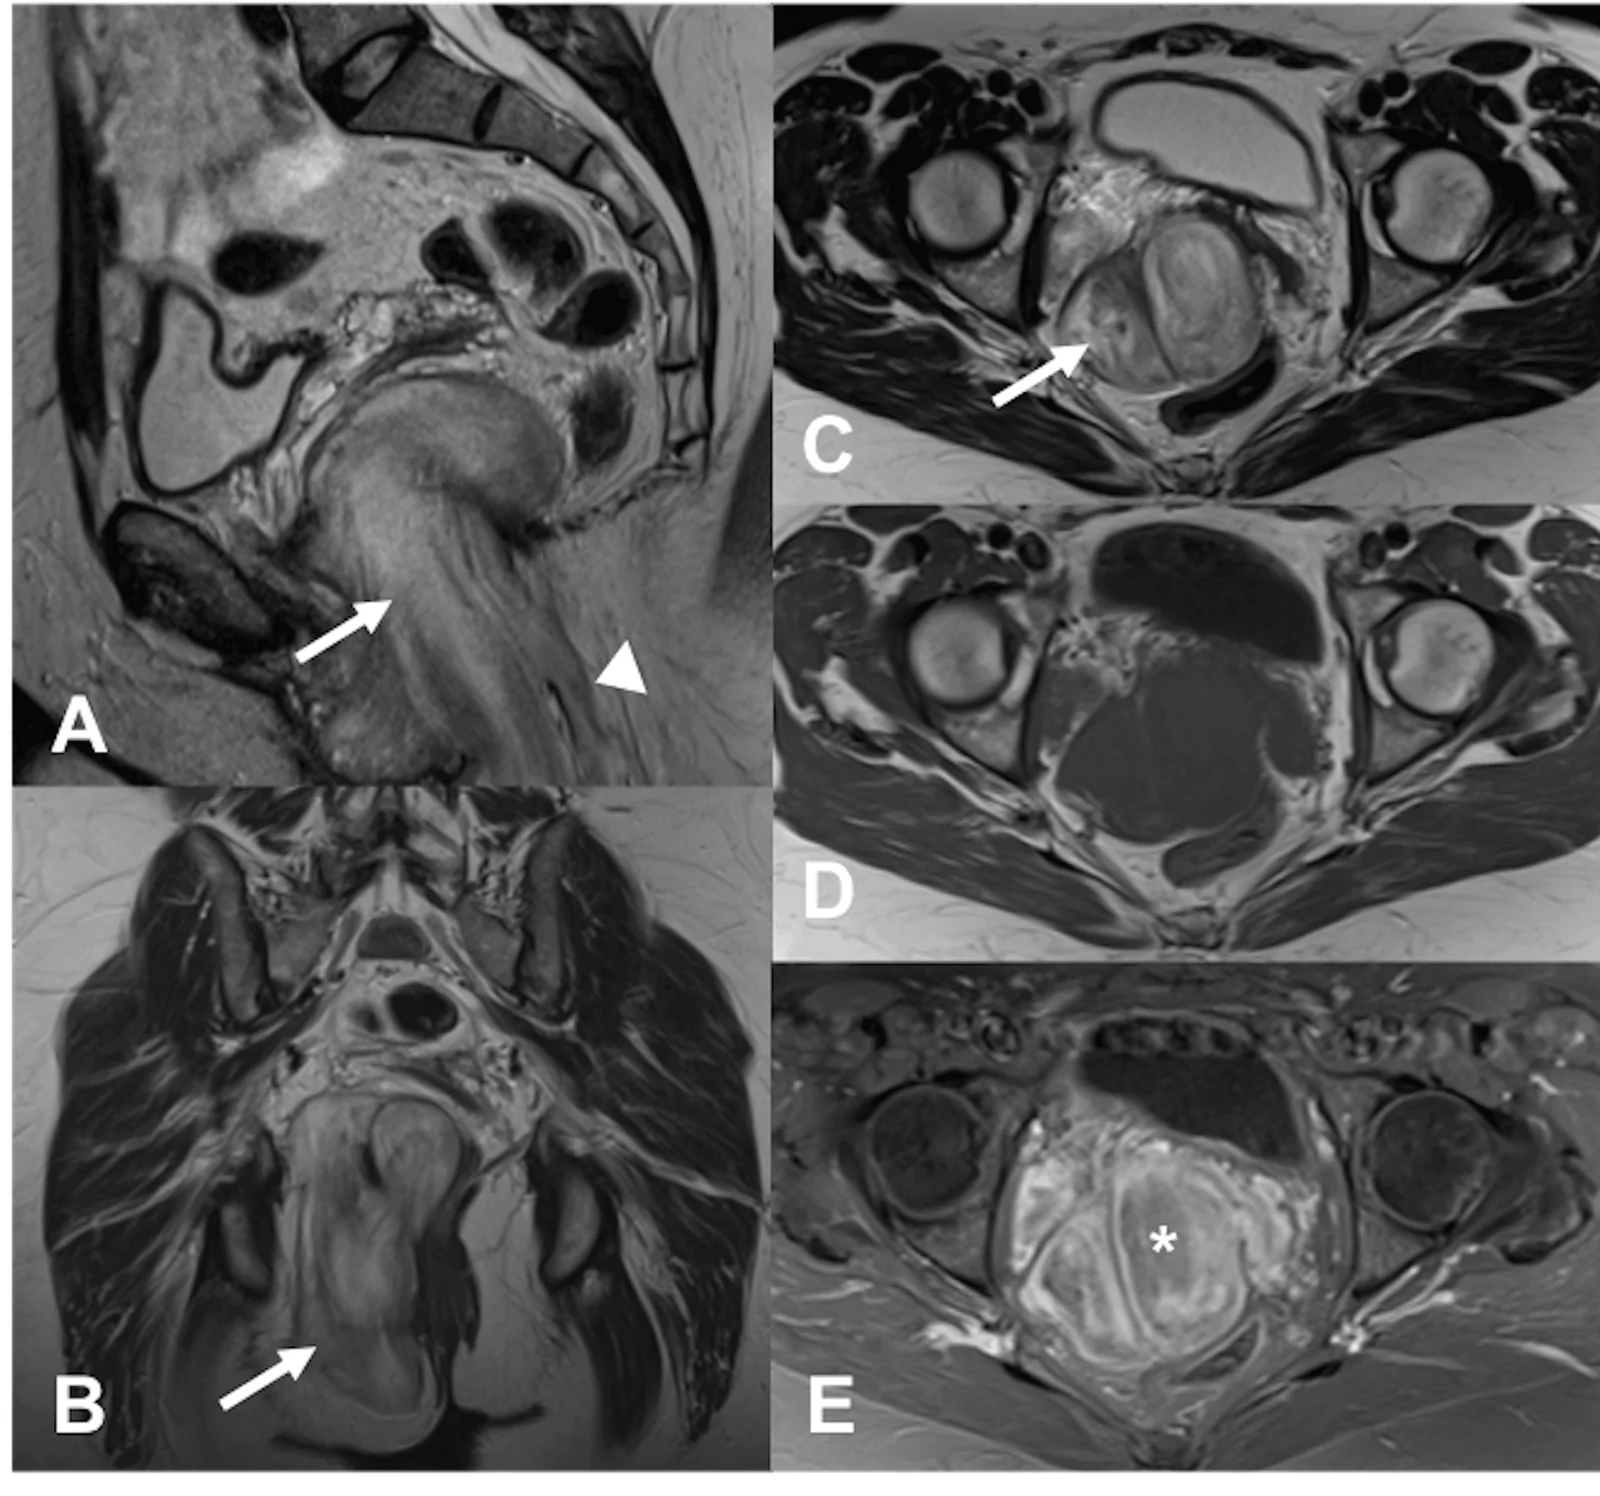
MRI of the pelvic tumor A–C) T2-weighted images in the sagittal (A), coronal (B), and axial (C) planes demonstrate a multilobulated mass centered in the rectovaginal space and right ischiorectal fossa, extending into the intergluteal cleft, with infiltrative finger-like projections into the surrounding soft tissues (arrowhead in A), measuring 93 × 67 × 124 mm (transverse × anteroposterior × craniocaudal). The mass demonstrates high signal intensity with a laminated appearance (arrows). D) Axial unenhanced T1-weighted imaging demonstrates isointense signal relative to skeletal muscle. E) Axial postcontrast fat-suppressed T1-weighted imaging demonstrates intense heterogeneous enhancement with a swirling pattern (*).
